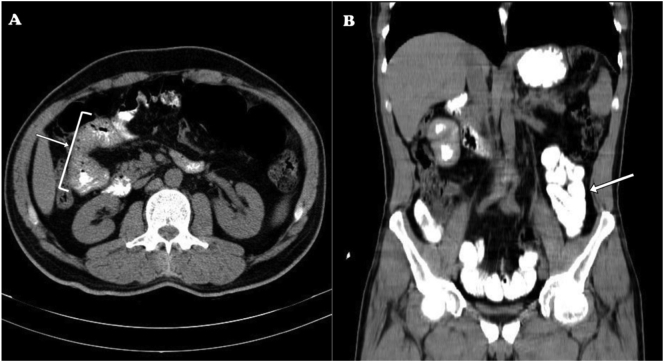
Patient's contrast-enhanced abdominopelvic CT scan, axial view (A) and coronal view (B); arrows indicate contrast-highlighted intestinal dilation filled with food impaction, suggesting the presence of a bowel obstruction.
